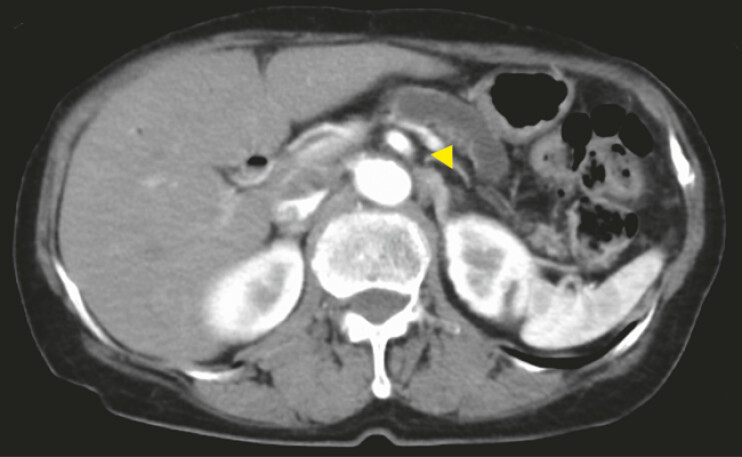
Computed tomography shows the dilation of the main pancreatic duct (yellow arrowhead).
